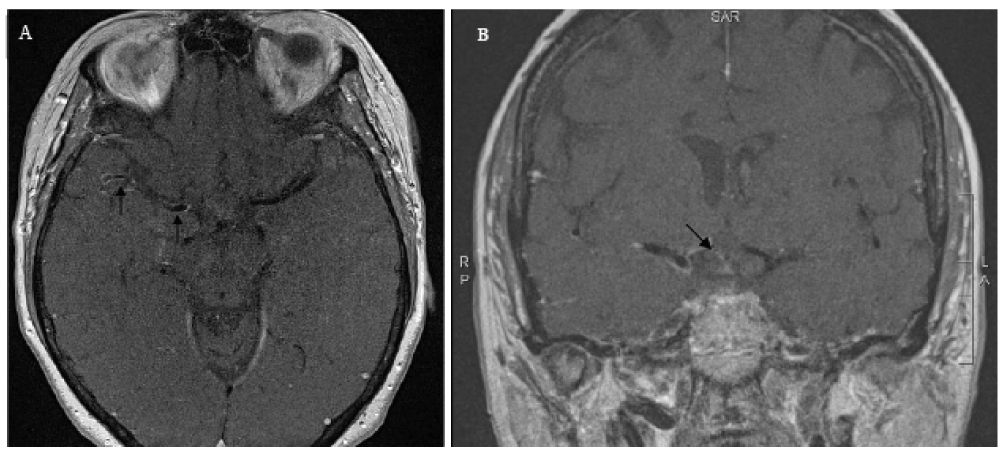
Black-blood MRI demonstrates unilateral enhancement. (A) Post-contrast black blood MRI images demonstrate enhancement of the right MCA (black arrows) and (B) of the right ACA (black arrow).
